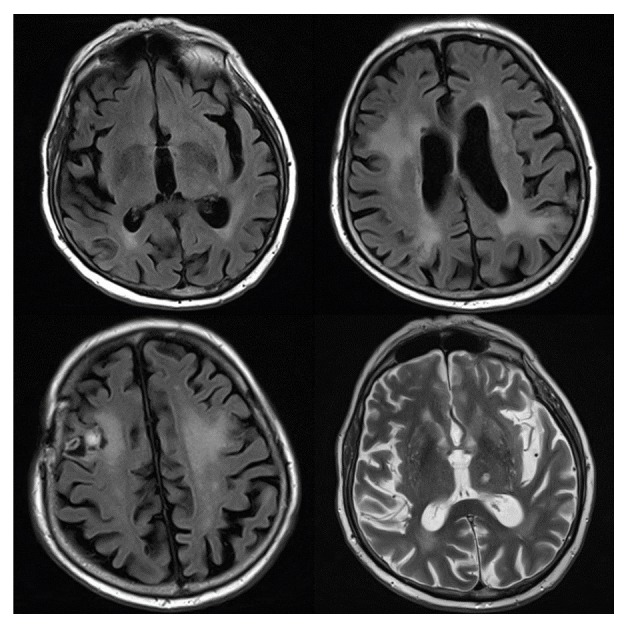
MRI at six-month posttherapy follow-up (day 270). Many of the areas previously demonstrating diffusion hyperintensity have improved and/or resolved. Several areas of diffusion hyperintensity remain. FLAIR hyperintensities are grossly similar except for some improvement in the left thalamic region and some worsening in the right parietal region.
